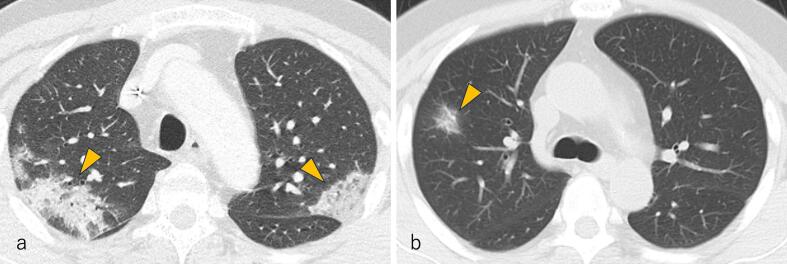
Pre- and postoperative thoracic CT findings. (a) Computed tomography (CT) on postoperative day 5 revealed new ground-glass opacity (GGO) in both lungs(arrowheads). (b) CT performed one day prior to surgery showing a new GGO in the right upper lobe (arrowhead). GGO: Ground-glass opacity CT: Computed tomography.
